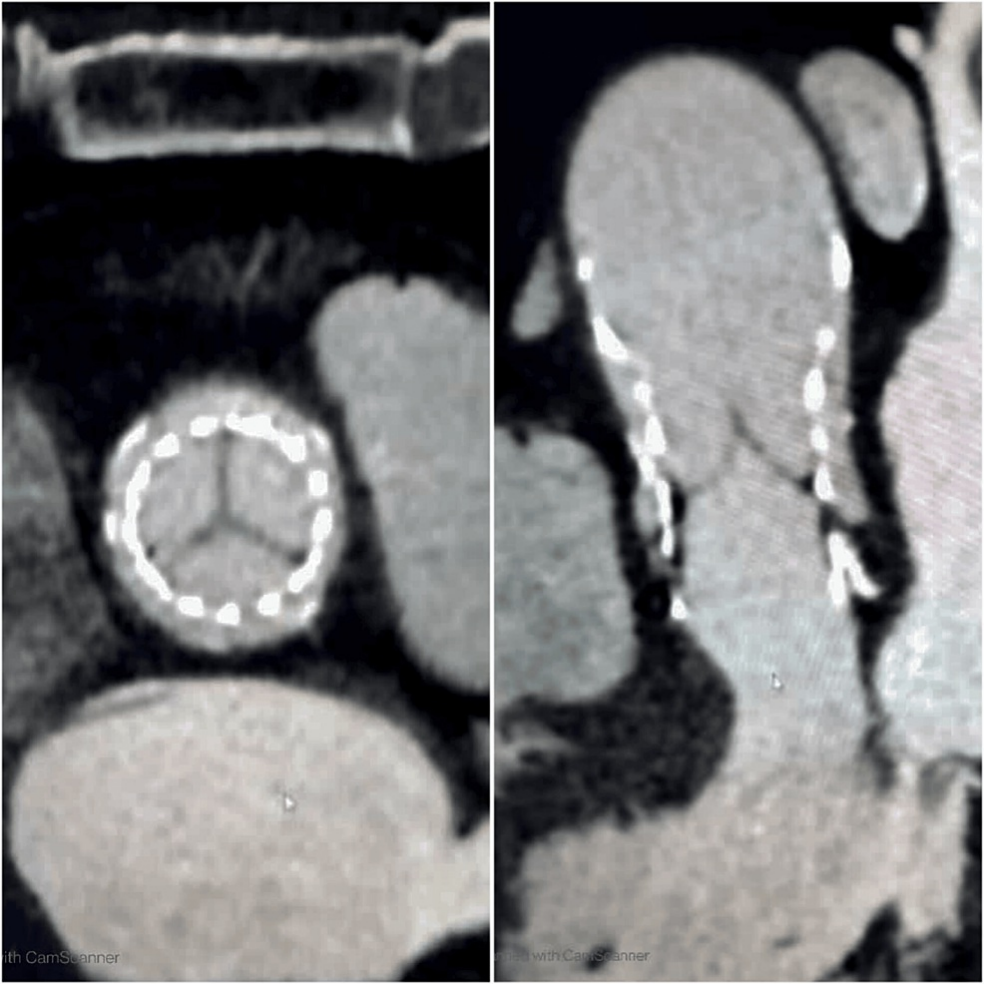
Repeat cardiac CT after three months showing resolution of HALT in short and long axes CT, computed tomography; HALT, hypoattenuated leaflet thickening
